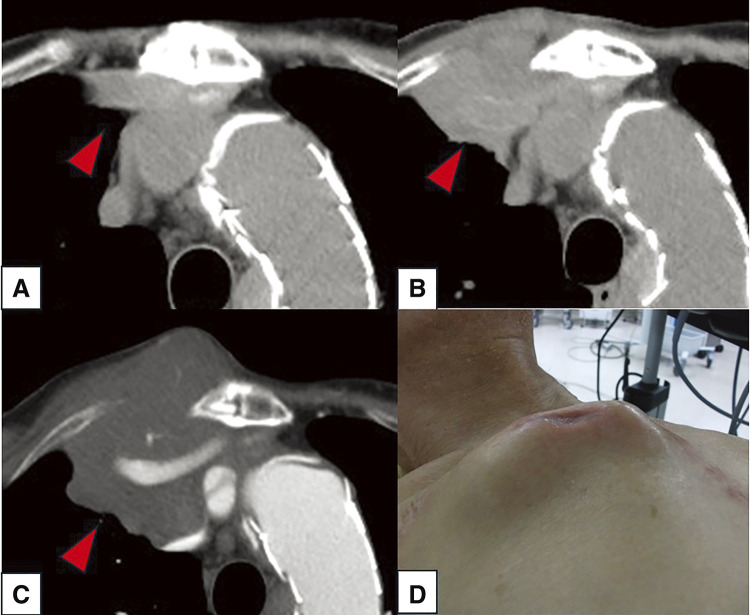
Fig. 2 Changes in the mass on preoperative computed tomography scan. The red arrowhead indicates the enlargement of the anterior mediastinal mass during this course. (A) 13 months before admission, (B) 1 month before admission, (C) current admission, and (D) preoperative external findings.
